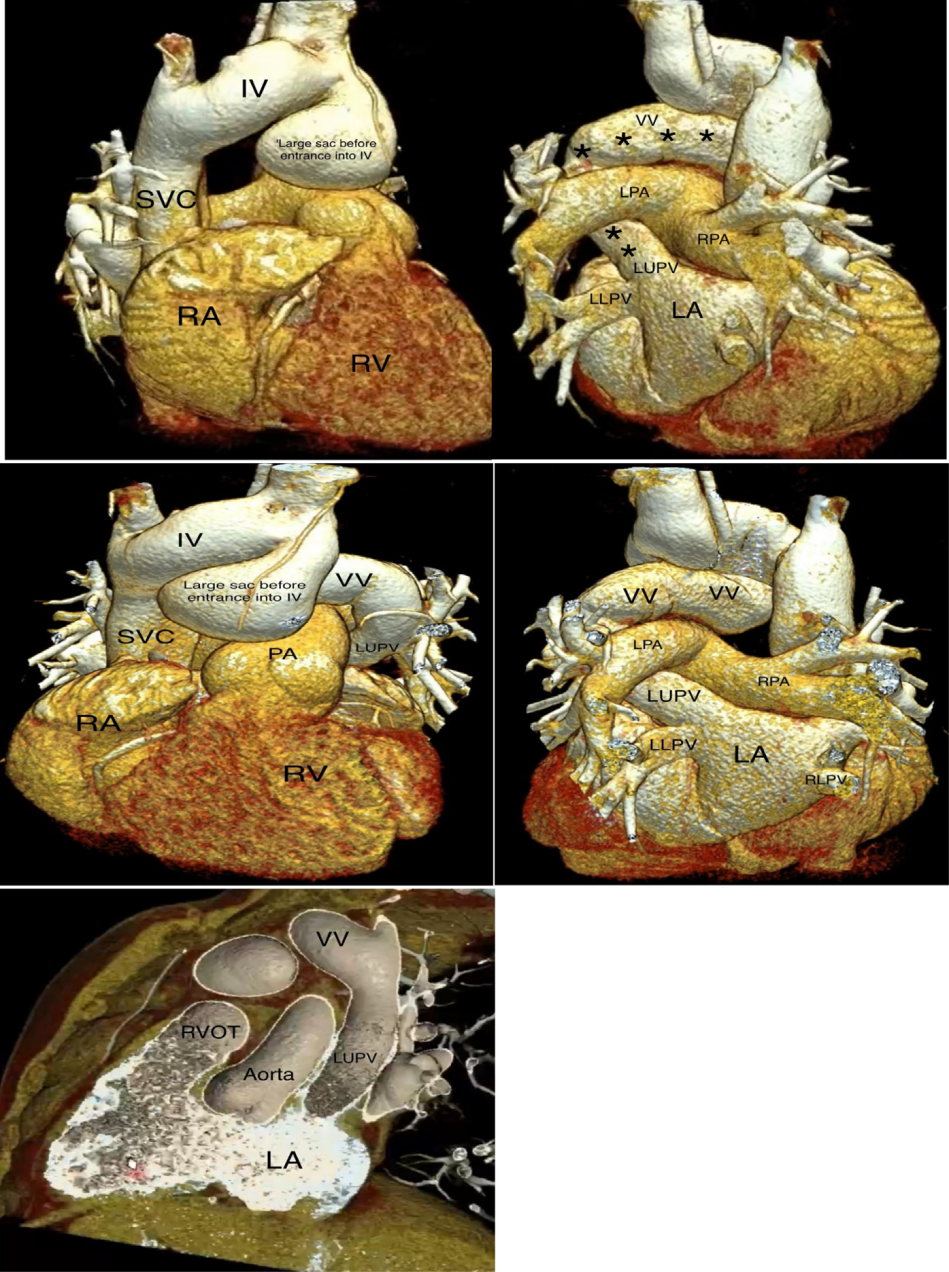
3-Dimensional Volume-Rendered Reconstruction Images Three-dimensional volume-rendered reconstruction images of cardiac computed tomography demonstrated the LUPV with a double drainage into the LA and dilated tortuous VV (solid stars show the track connection of LUPV and VV) that directed to a large sac before entrance into the IV and then the SVC. LLPV = left lower pulmonary vein; LP = left pulmonary artery; PA = pulmonary artery; RLPV = right lower pulmonary vein; RPA = right pulmonary artery; RVOT = right ventricular outflow tract; other abbreviations as in Figures 2 and 3.
